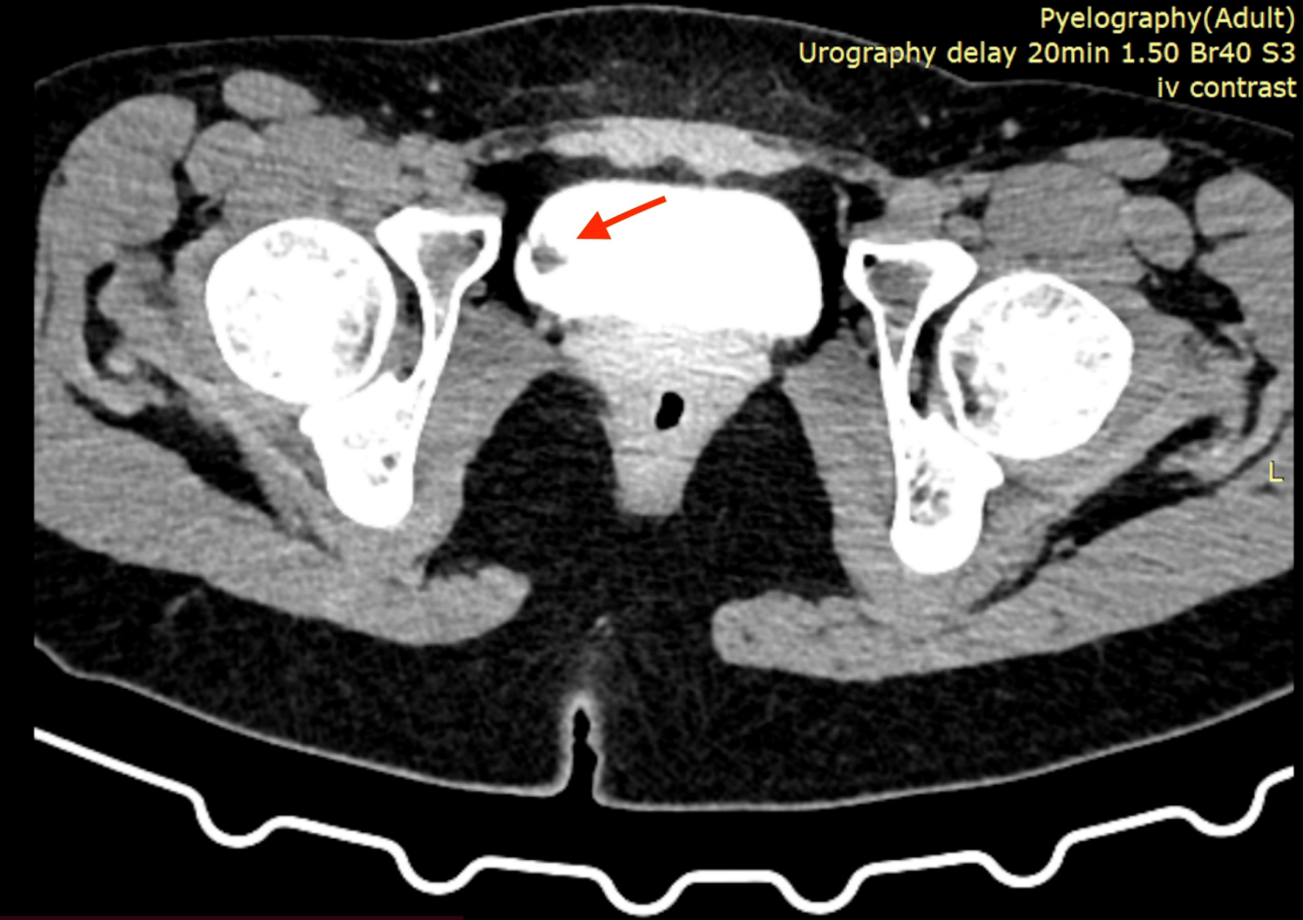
CTU showing a solitary papillomatous lesion at the right lateral bladder wall. CTU, computed tomography urography.
